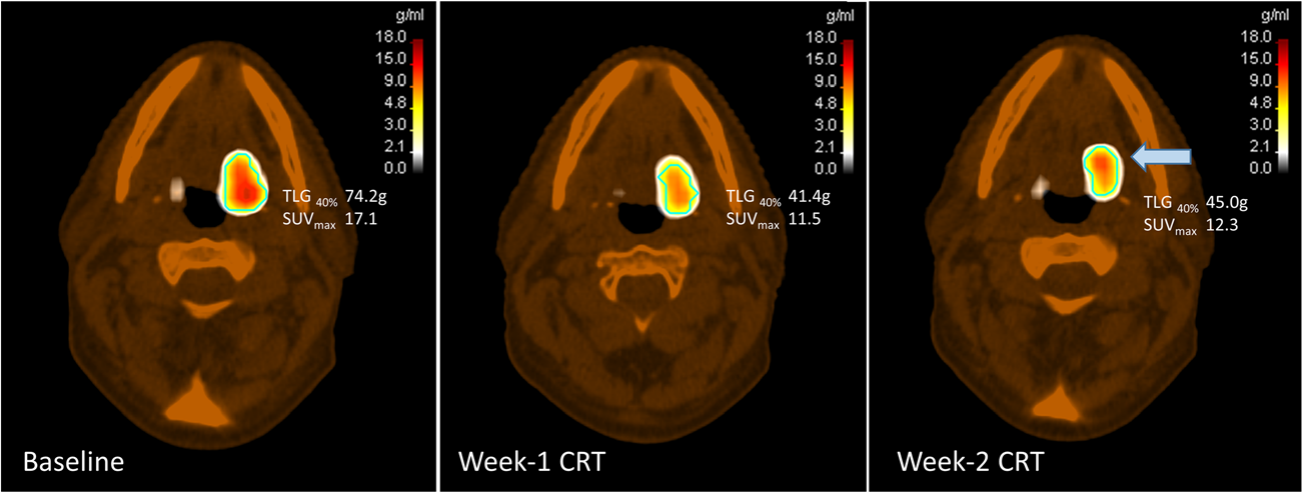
An example of a responder having a paradoxical increase in primary tumor SUV at week 2 (highlighted by the blue arrow, cyan contour = MTV_40%_) despite a marked initial decrease at week 1. This phenomenon was observed in a few other responders, which may be confounded by radiotherapy-induced peritumoral inflammation with cumulative fractions
